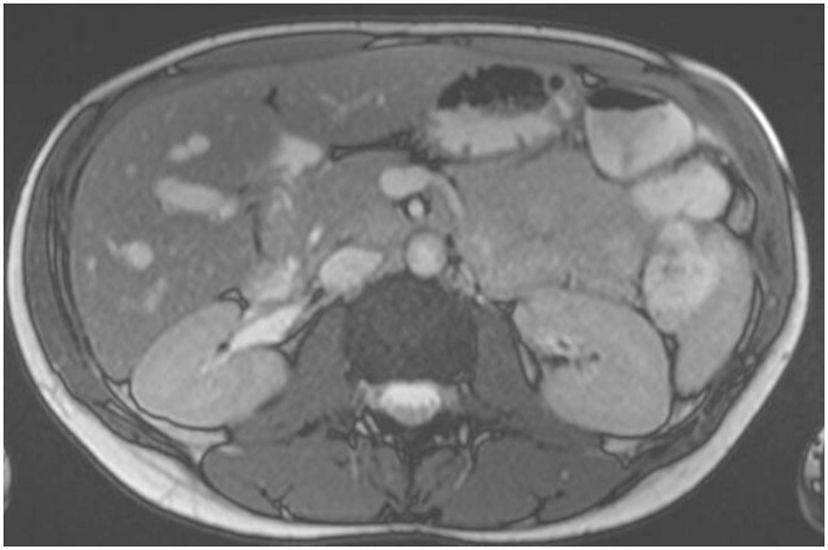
MRI showing complete response of liver metastases from choroidal melanoma.
